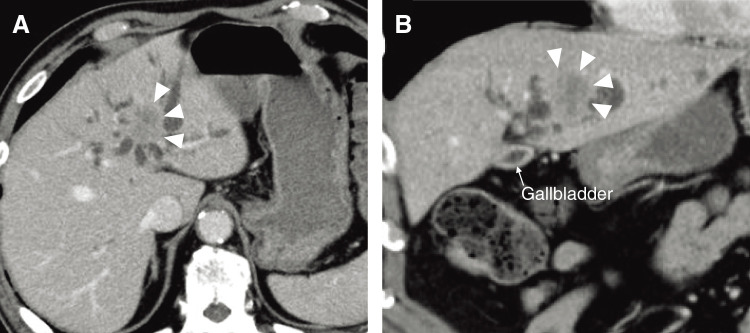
Contrast-enhanced computed tomographic images of the patient. (A) Axial section image. (B) Coronal section image. Dilatation of the intrahepatic bile ducts, a tumorous lesion, and a hypovascular tumor of 30 mm in size in the left lateral segment of the liver (arrowheads).
